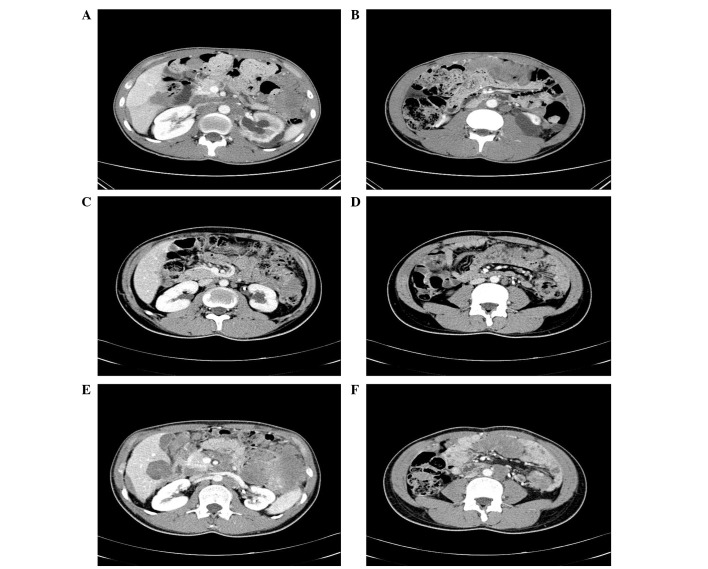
Abdomen and pelvis computed tomography prior to and following treatment. (A and B) Conglomerated lymph nodes (LNs) were observed along the aorta and inferior vena cava, as well as in the mesentery. Hydronephrosis of the left kidney and peritoneal seeding were also observed. (C and D) Conglomerated LNs and the peritoneal mass showed a decrease in size and the hydronephrosis of the left kidney had improved following the second cycle of chemotherapy. (E and F) Disease progression was observed following completion of chemotherapy.
